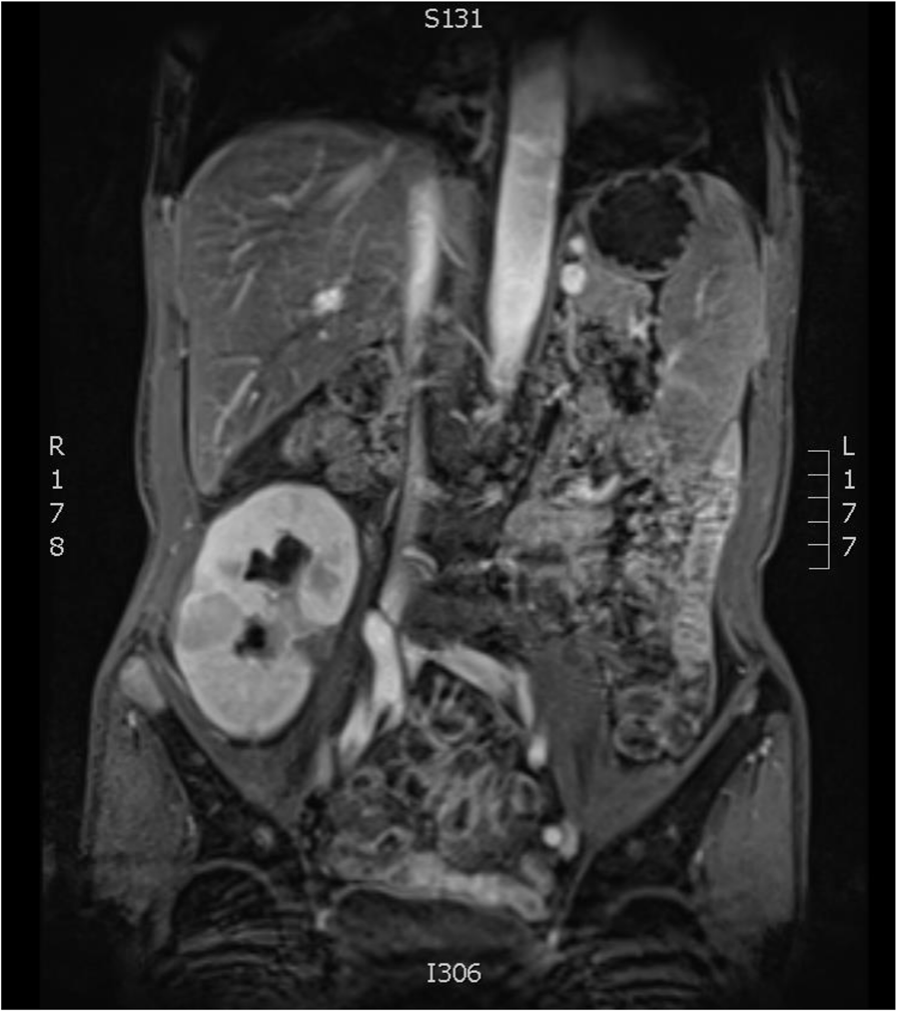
Enhanced MRI demonstrated multiple, variably sized quasi-circular lesions with slightly short signal intensity on T1WI, slightly long signal intensity on T2WI, and high signal intensity on DWI. The larger size lesion was about 4.66 cm by 3.10 cm
